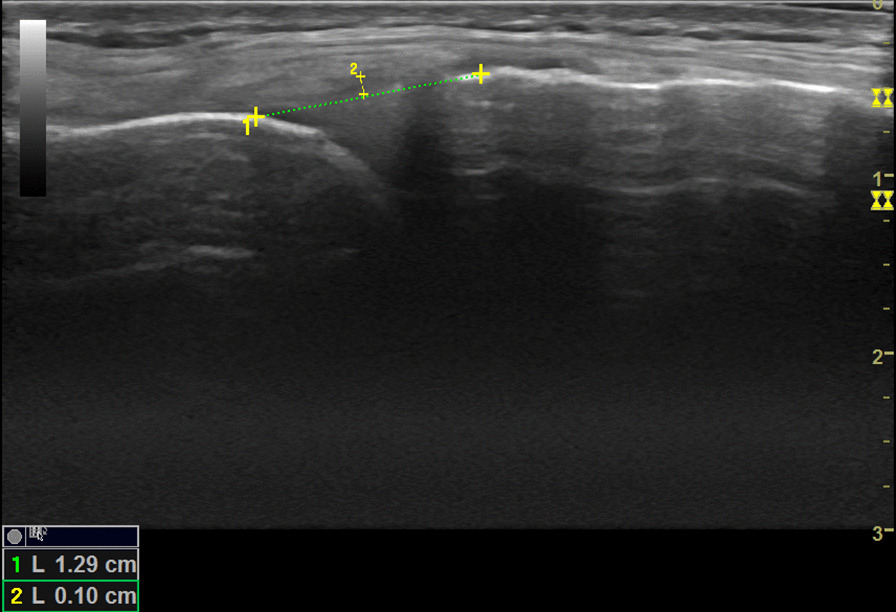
Sonography of the medial meniscus while knee in 20° flexion with internal rotation
